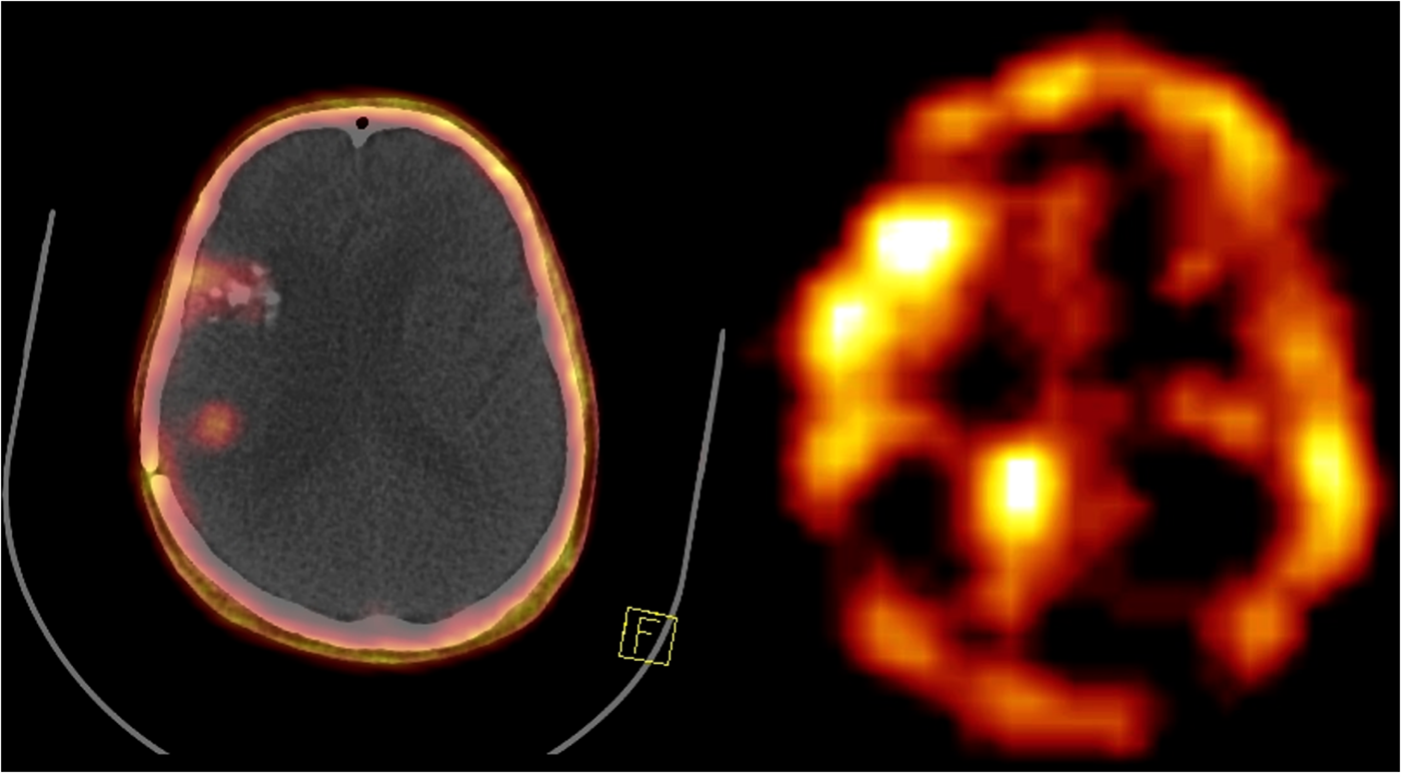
A 55-year-old man with recurrent high-grade glioma in the right temporoparietal region underwent ^68^Ga-FAPI PET/CT showing three cerebral lesions in the right frontal and right temporoparietal regions (left side). The patient received one cycle of ^177^Lu-FAPI (right side).
